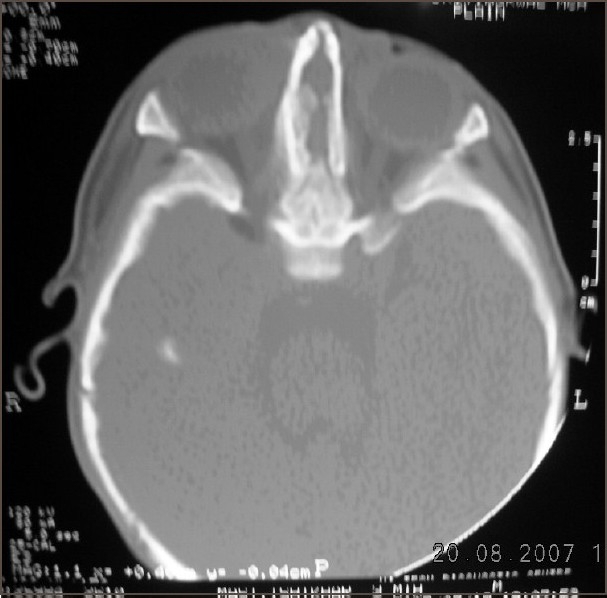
CT scan showing narrow nasal cavity with pyriform aperture and choanal atresia
